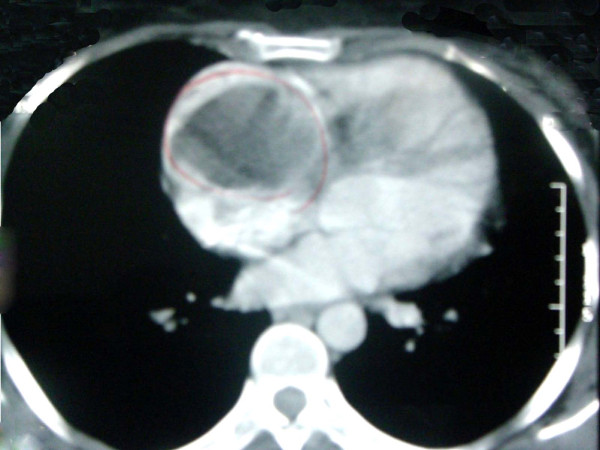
An image of a chest computed tomography scan with intravenous contrast shows an enlarged cardiac silhouette with intra-atrial hypodensity.
